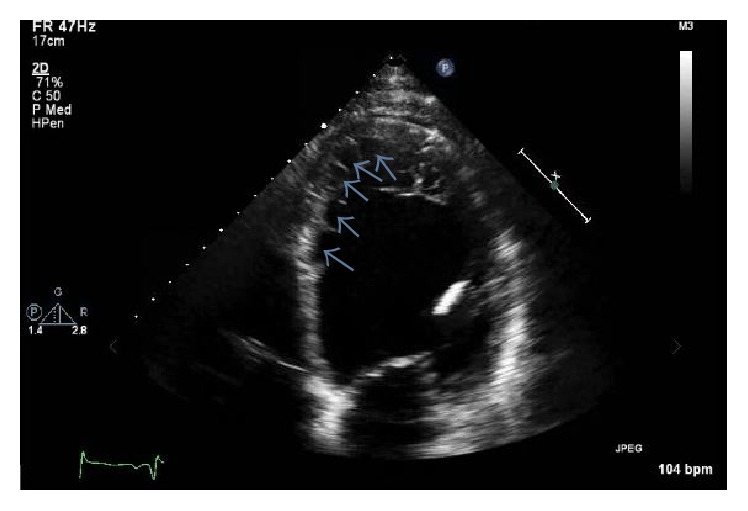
Two-dimensional echocardiogram in our patient demonstrating prominent trabeculations and deep intratrabecular recesses (marked by arrows).
